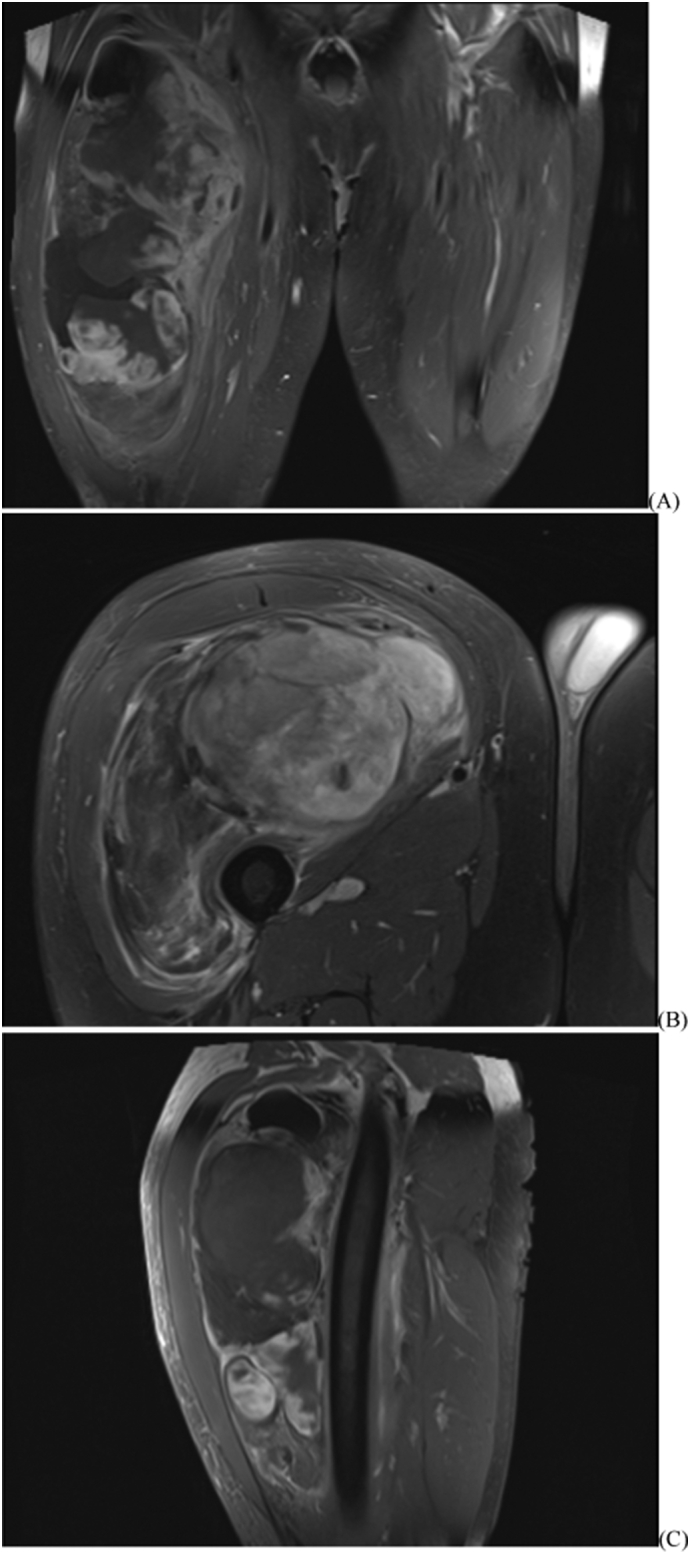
Patient 1 post-contrast MRI of right thigh tumor. (A) T1 fat-saturated coronal; (B) proton density fat-saturated axial; (C) T1 fat-saturated sagittal.
